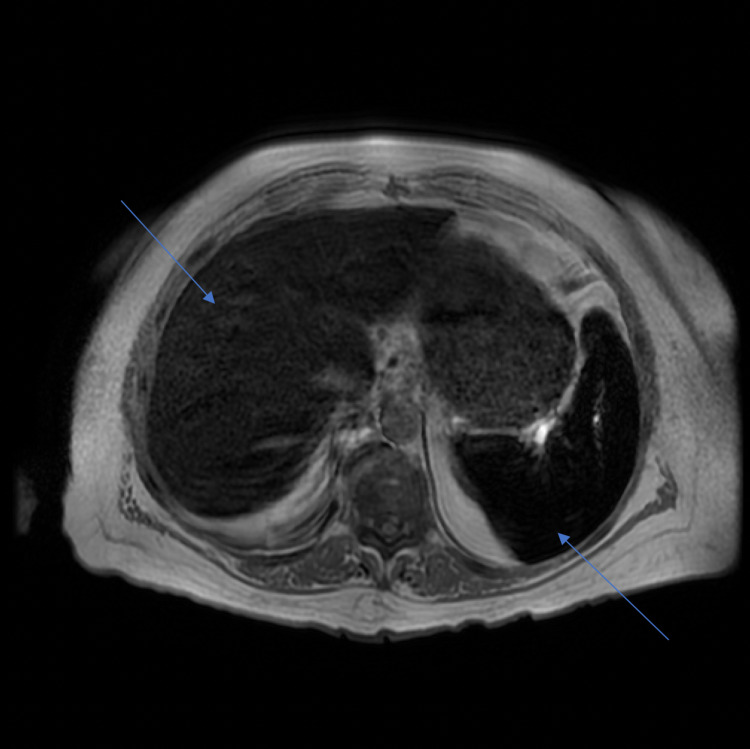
MRI of the abdomen showing iron overload in the liver and spleen. The calculated iron concentration in the liver measured 148 µmol/g (normal iron concentration in the liver is less than 36 µmol/g). MRI: magnetic resonance imaging
